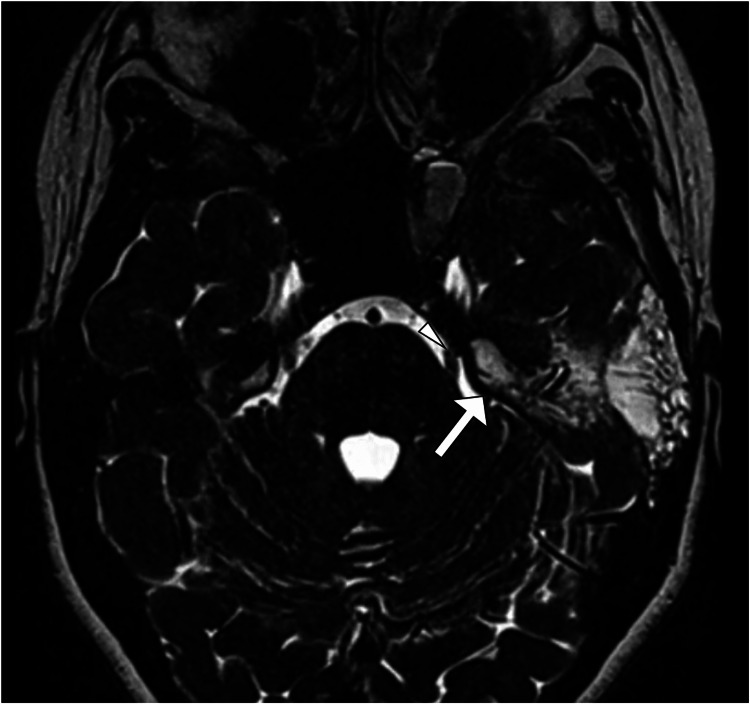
MRI of the brain in the axial plane using the CISS sequence, revealing the lesion of the petrous bone (arrow) in close proximity to the left trigeminal nerve (arrowhead), which can explain the patient's symptoms. MRI, magnetic resonance imaging; CISS, constructive interference in steady state
